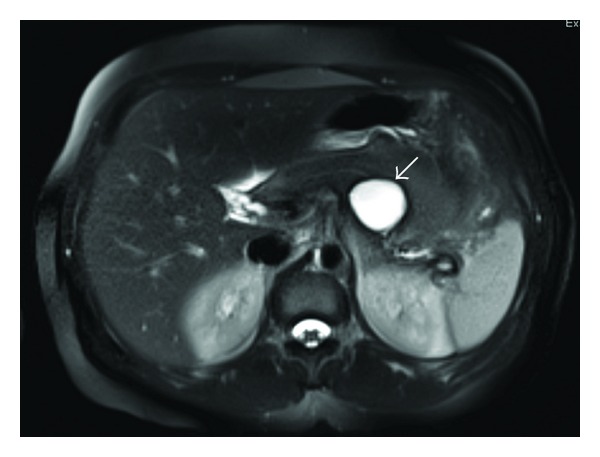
Mucinous cystic neoplasm (arrow) on MRI abdomen.
